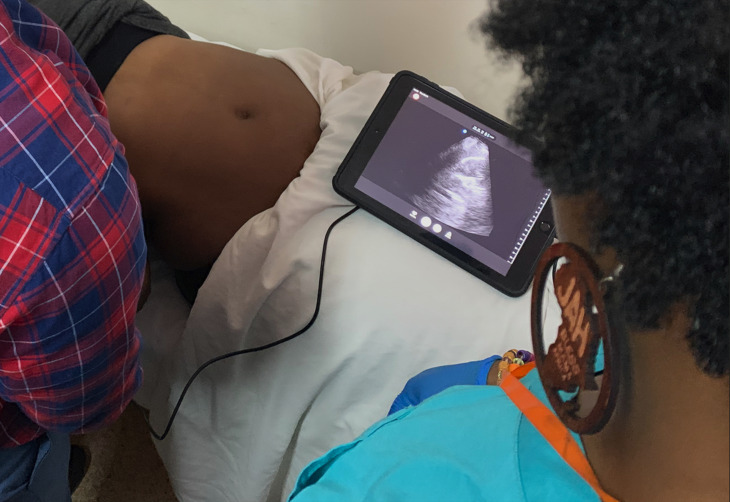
Use of point of care ultrasound (POCUS) for evaluation of a patient with abdominal pain.
